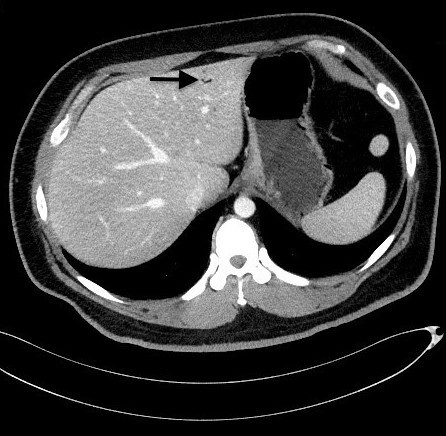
Computed tomography with contrast of abdomen shows hepatic portal venous gas in the anterior portion of the left hepatic lobe (arrow).
